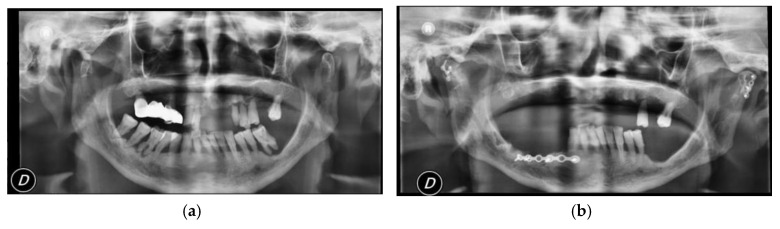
(a) Pre-operatory panoramic X-ray; (b) post-operatory panoramic X-ray of patient #3.
